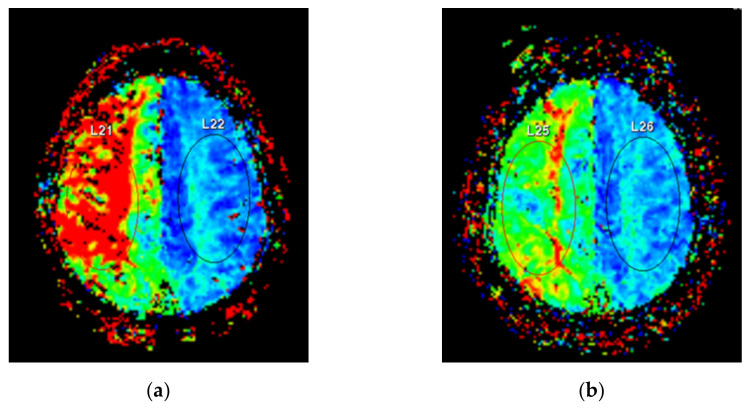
Perfusion weighted magnetic resonance imaging. Regions of interest were located at bilateral centrum semiovale areas. (a) Preoperative imaging reveals delayed time to peak at the right subcortical area. (b) Postoperative imaging shows improved cerebral perfusion with decreased color difference between the affected and unaffected sides.
